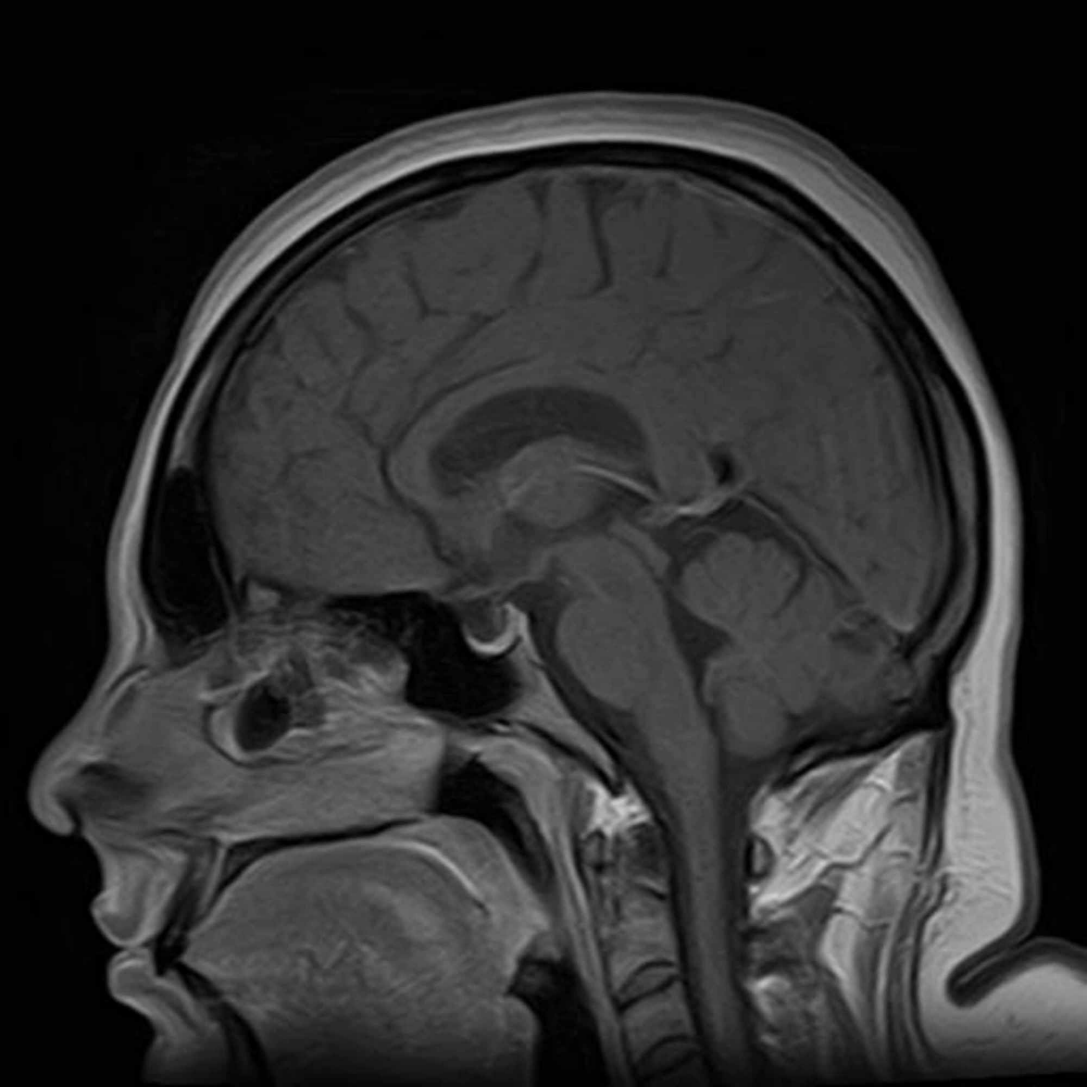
MRI brain with contrast (sagittal view) MRI = Magnetic Resonance Imaging
